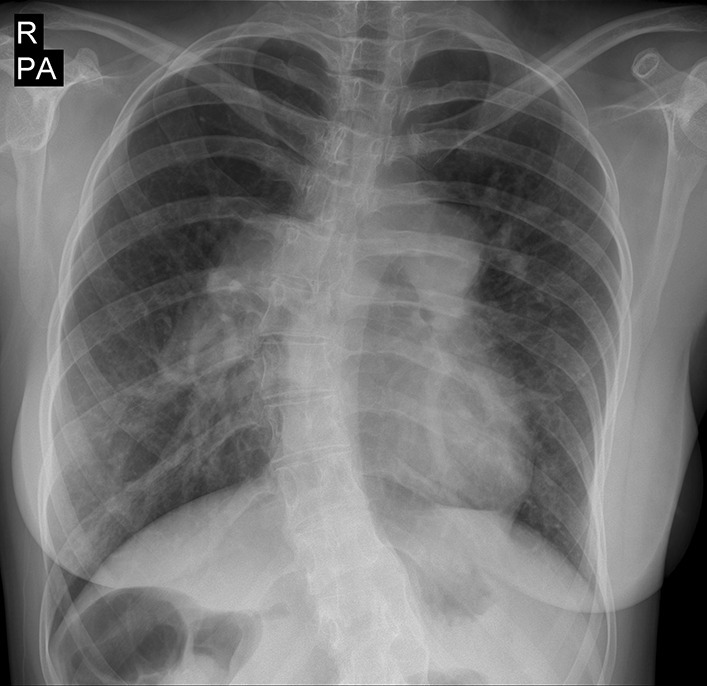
Chest X-ray showing pulmonary artery dilation, right ventricular enlargement and scoliosis
